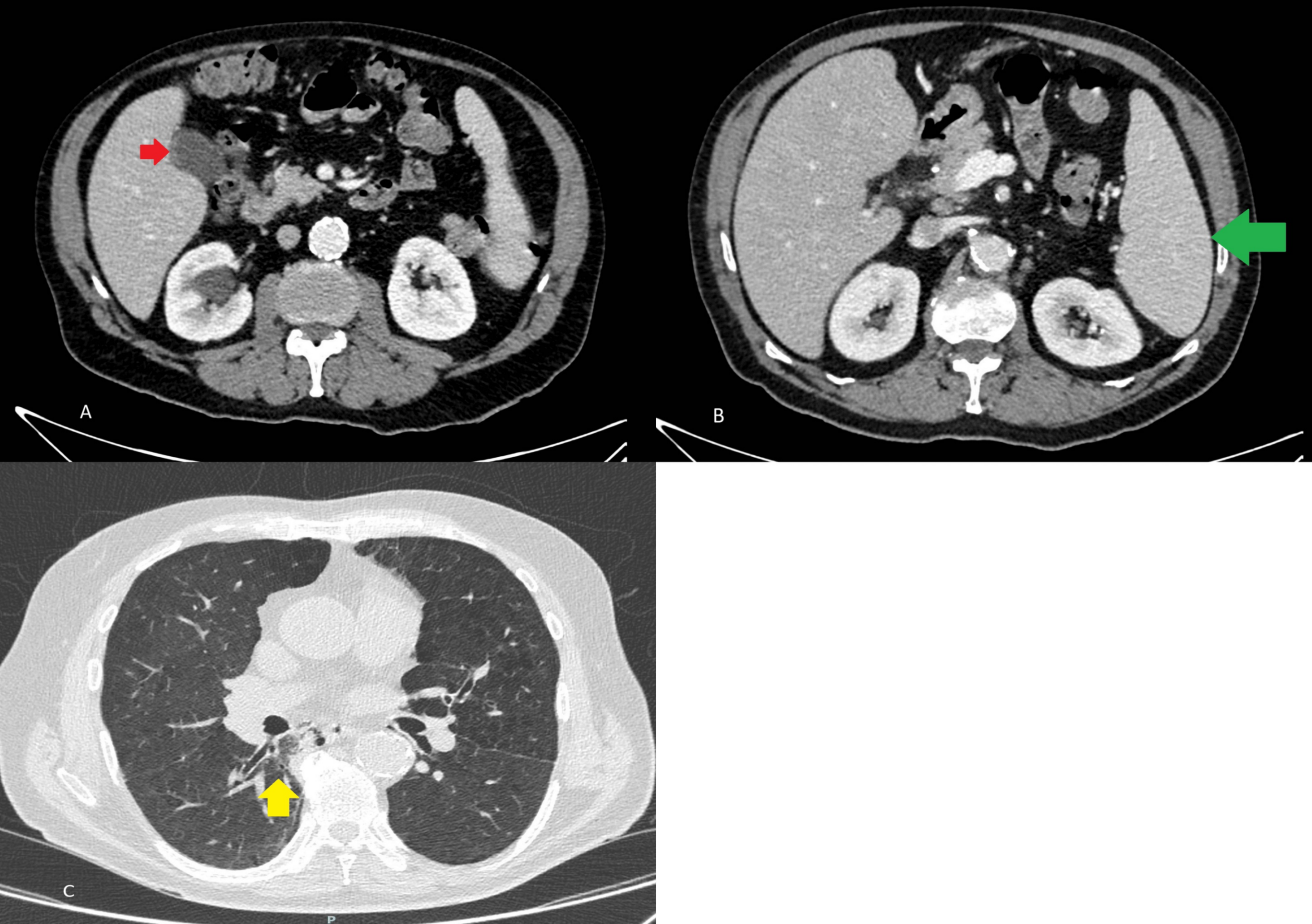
Contrast-enhanced CT of the abdomen A and B: Axial sections of the venous phase show edema of the gallbladder wall without signs of cholecystitis (red arrow) and splenomegaly (green arrow); C: The thorax axial section shows consolidation of the right lower lobe (yellow arrow)
